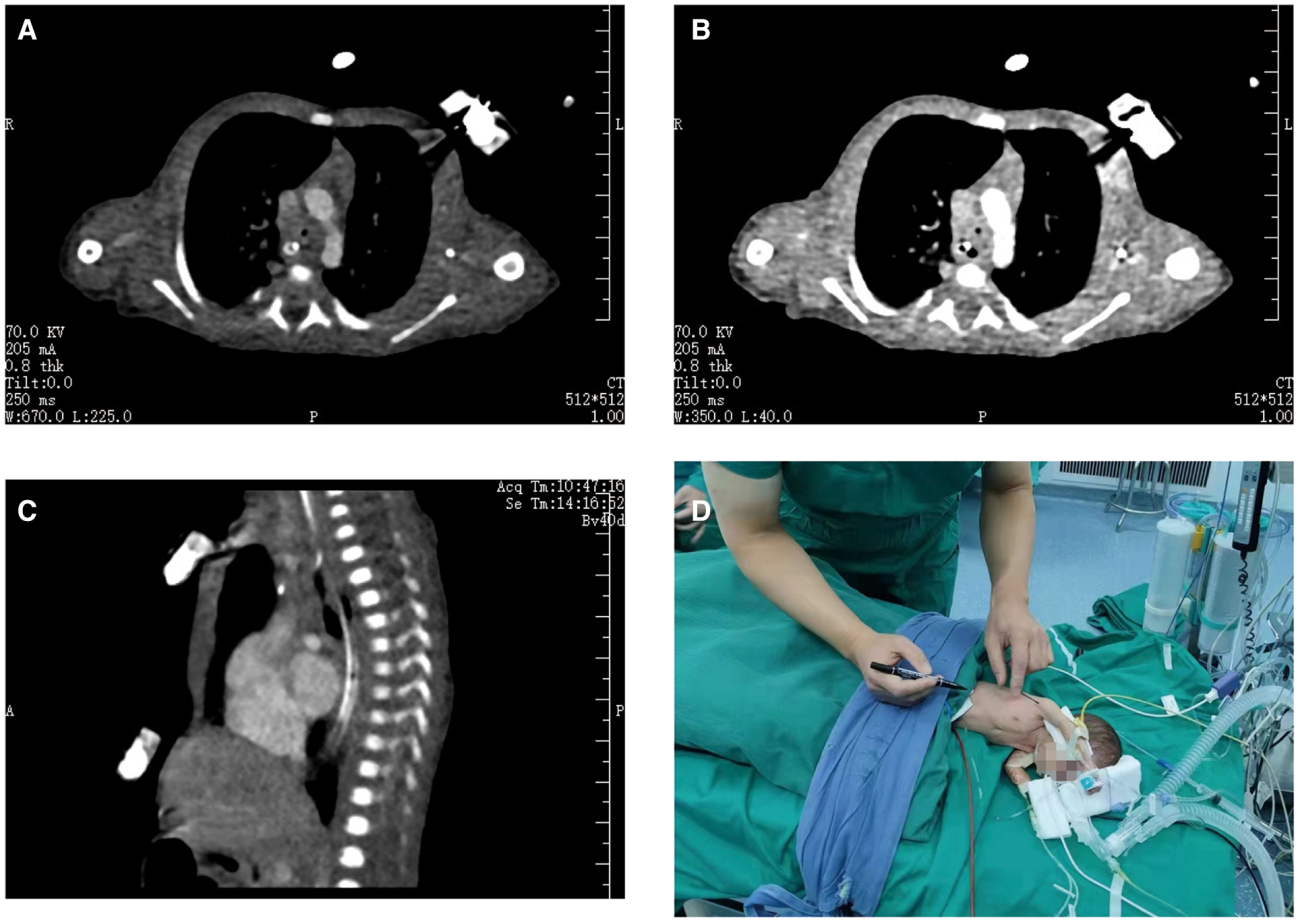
Preoperative examination of the patient. A computed tomography scan of his chest revealed that the lumen above the tracheal carina was narrow, with a diameter of approximately 1.7 mm at its narrowest point and involvement of approximately 7 mm in length (A–C). We were going to operate on the infant with ECMO-assisted tracheal resection and end-to-end anastomosis (D).
